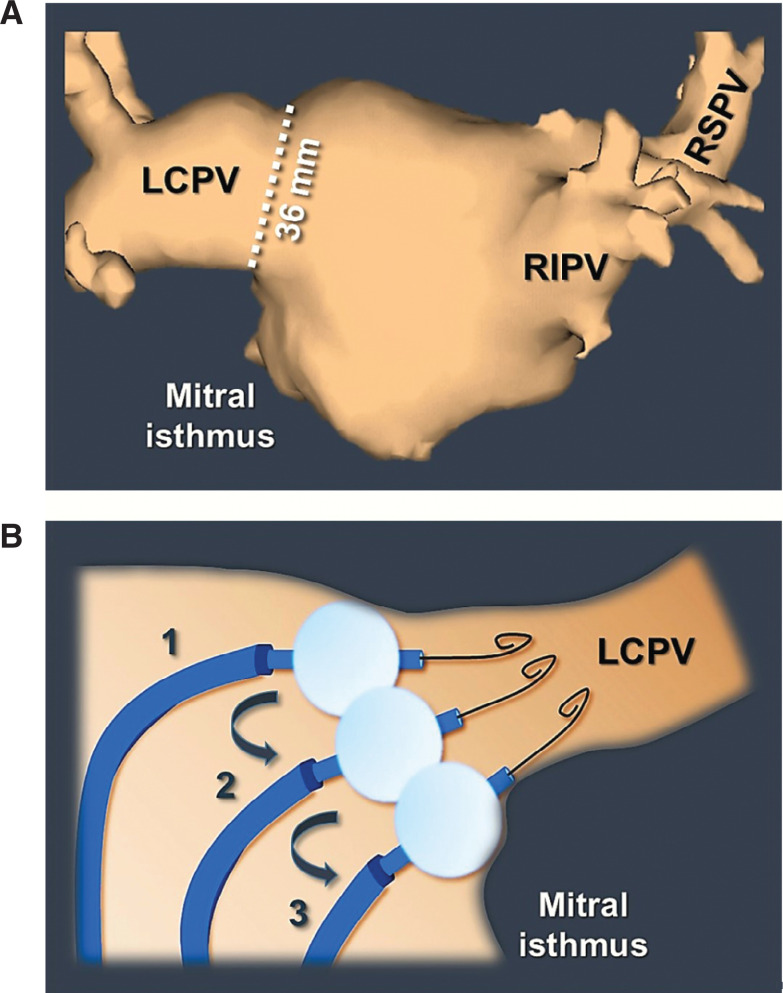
Non-PV occlusive cryoballoon maneuvers for isolation of large-sized PVs. A: A cardiac computed tomography image demonstrating a left common PV with an ostium measuring 36 mm in diameter. B: When approaching such a vein using a 28-mm cryoballoon, the operator should target the PV with a series of nonocclusive applications to avoid a distal-level (ostial) PVI. In this manner, the PV is ablated antrally in a segmental fashion, as shown in steps 1 to 3. A similar strategy can also be adopted in general when treating large-sized PVs, even in the absence of a “true” common PV ostium. LCPV: large-ostia left common pulmonary vein; RIPV: right inferior pulmonary vein; RSPV: right superior pulmonary vein.
